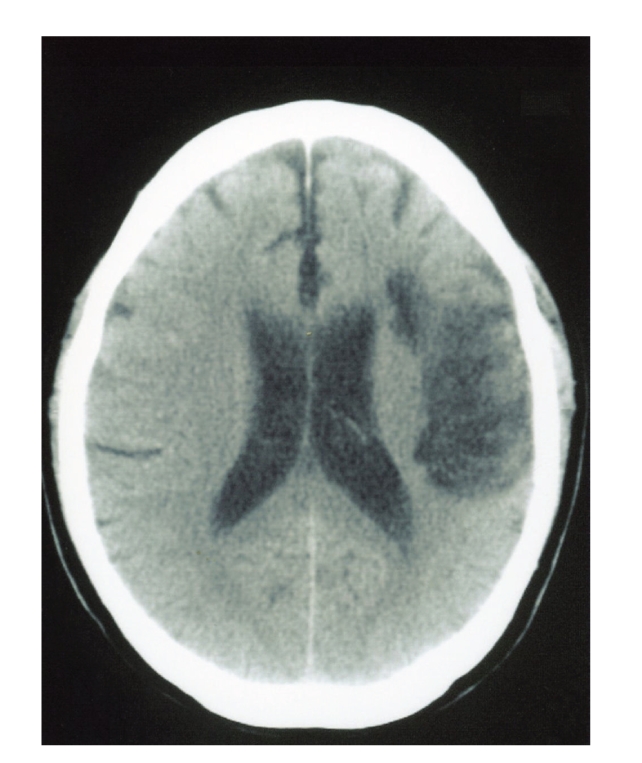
Axial Computed tomography of the brain demonstrated cortical infarction in the left frontal lobe.
